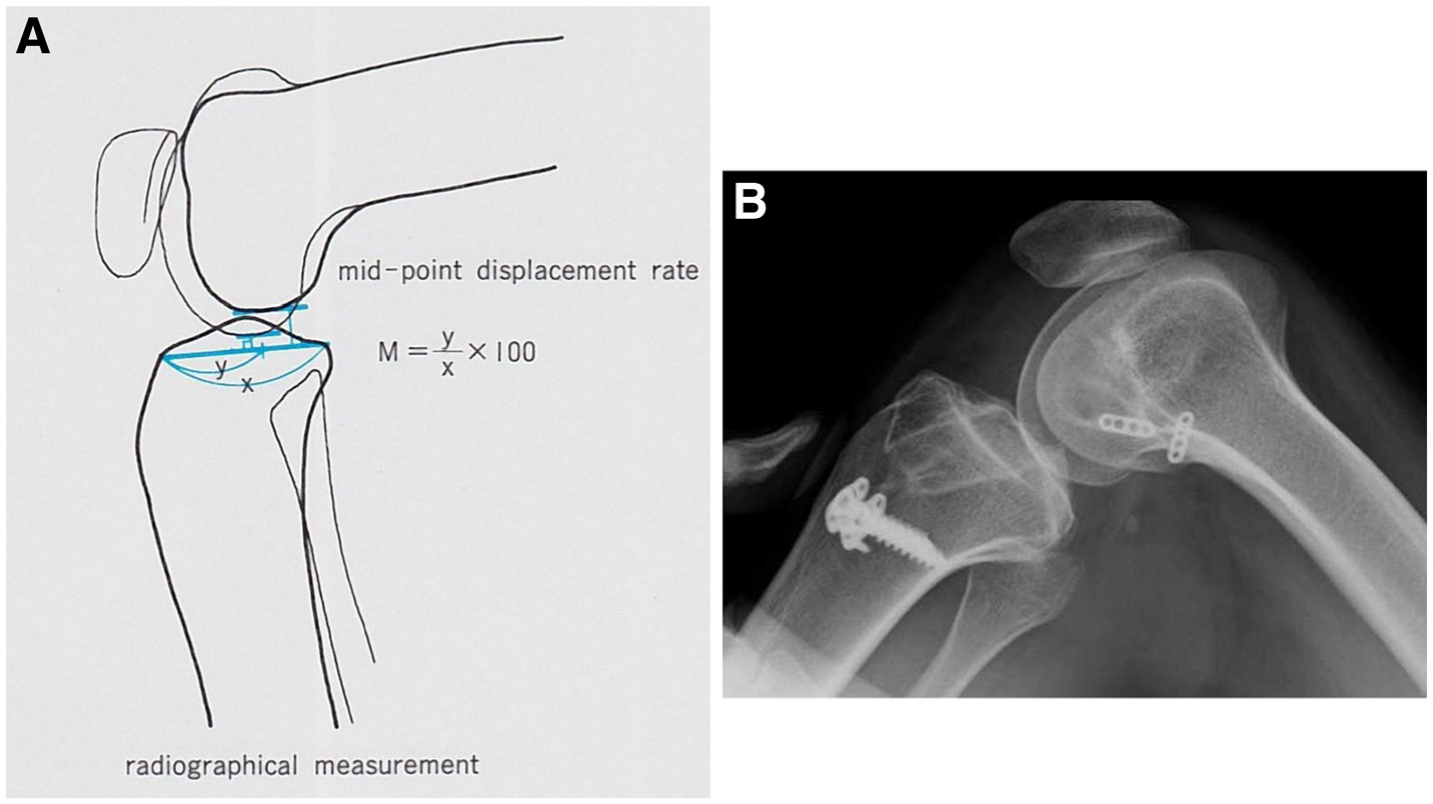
Stress X-ray findings. Stress radiography evaluated based on the results of midpoint measurements (A). A finding of greater than 70% of anterior tibial translation indicates ACL function failure. The results of the present case, at the six months postoperative evaluation revealed a 63% anterior tibial translation (B).
